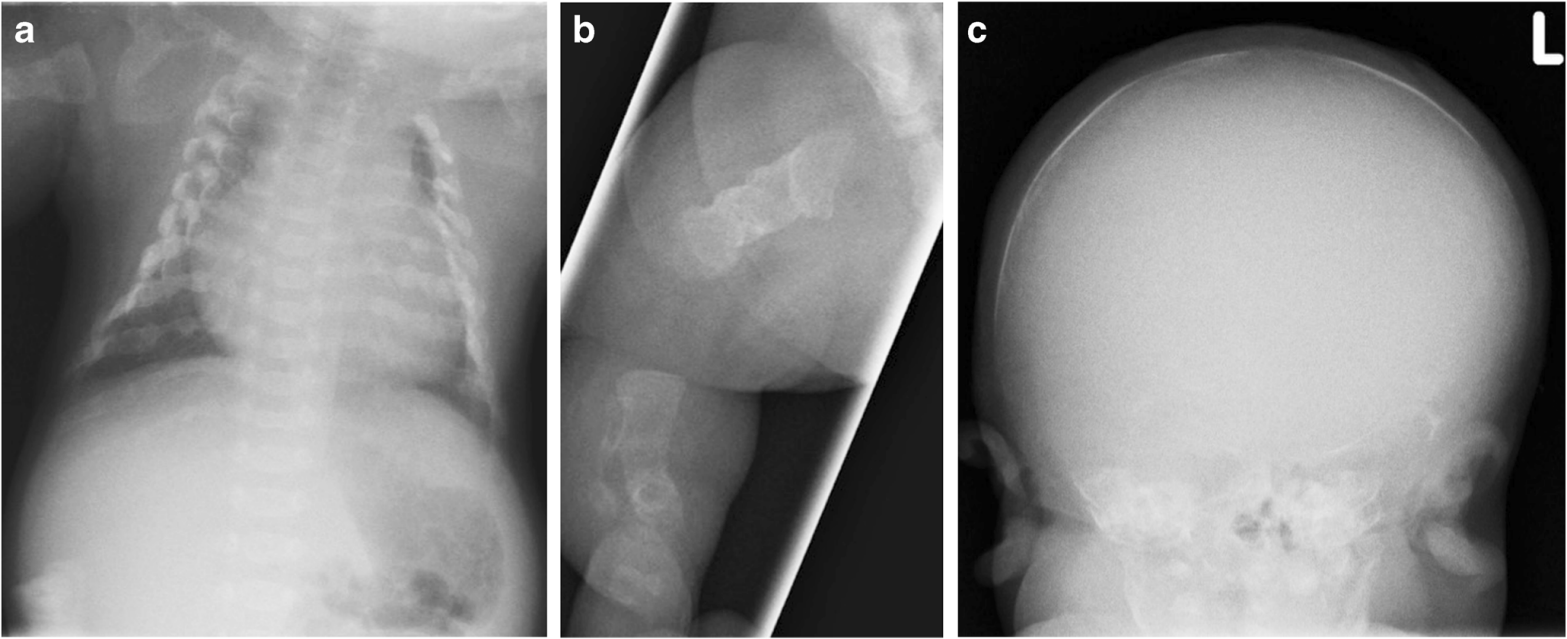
Radiographic features of an infant of unknown gender at birth (delivered at term) with severe osteogenesis imperfecta type III. a-c Anteroposterior radiographs show broad ribs with multiple fractures in the chest (a), broad tubular bones with multiple fractures of the right lower limb (b) and absent ossification of the skull vault (c)
